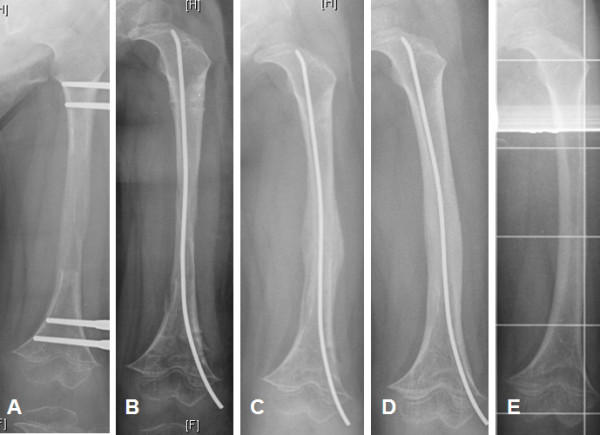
A-D Radiographic examination and diagnosis of a fracture in the regenerate during treatment. Boy (No 5 in Table 2) with femoral lengthening due to achondroplastic short stature, 5 years old: A) at the day of the removal of the fixator. B) day 0, after removal of the frame, one TEN was inserted due to prophylactic stabilization. C) x-ray at day 50 after removal shows a new callus formation within the regenerate, it must have been a fracture type 1b weeks ago, estimated caused by manipulation during insertion of the TEN, no other reason was applicable. D) 4 months (day 133) after removal of the fixator, before removal of the TEN with bony healing without loose of length or development of further malformation. E) late follow up, 1 year after removal of the TEN.
